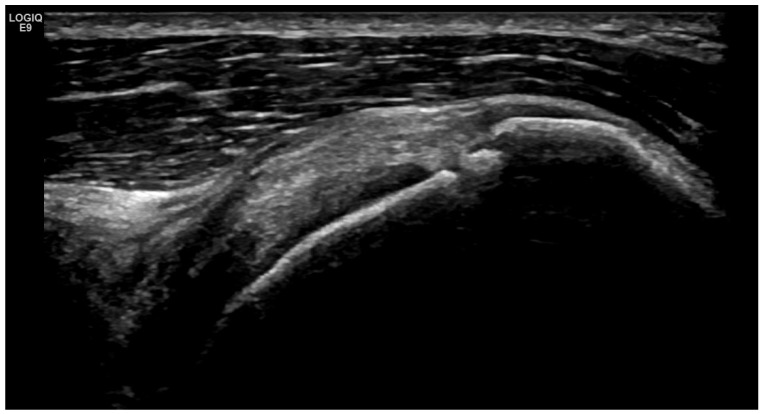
Ultrasound examination of a shoulder joint in a patient with omarthrosis. In parallel with the soft tissue changes at the level of the rotator cuff, a break in the bone contour is also found—a step-down defect corresponding to erosions.
